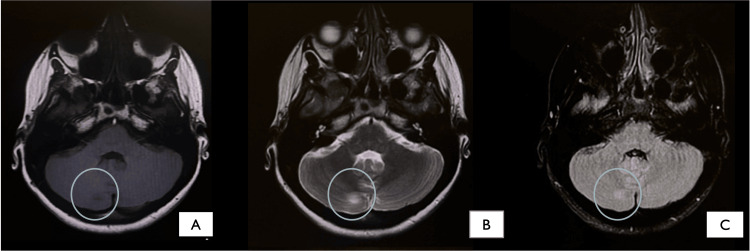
Regression of right cerebellar lesion seen on sequences: (A) T1, (B) T2, (C) FLAIR FLAIR: fluid-attenuated inversion recovery
